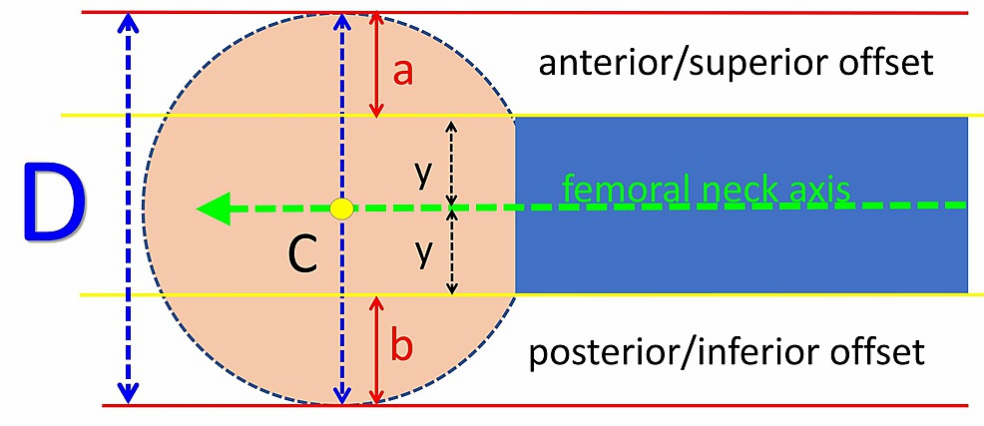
A hypothetical model of the normal femoral head-neck relation: The femoral head is considered a sphere and the femoral neck a cylinder. C: The center of the femoral head; D: the diameter of the femoral head (blue-dotted arrow); green arrow: the femoral neck axis; a: anterior/superior head-neck offset on the frog-lateral/anteroposterior pelvis x-ray, respectively; b: posterior/inferior head-neck offset on the frog-lateral/anteroposterior pelvis x-ray, respectively; y: the radius of the femoral neck.
